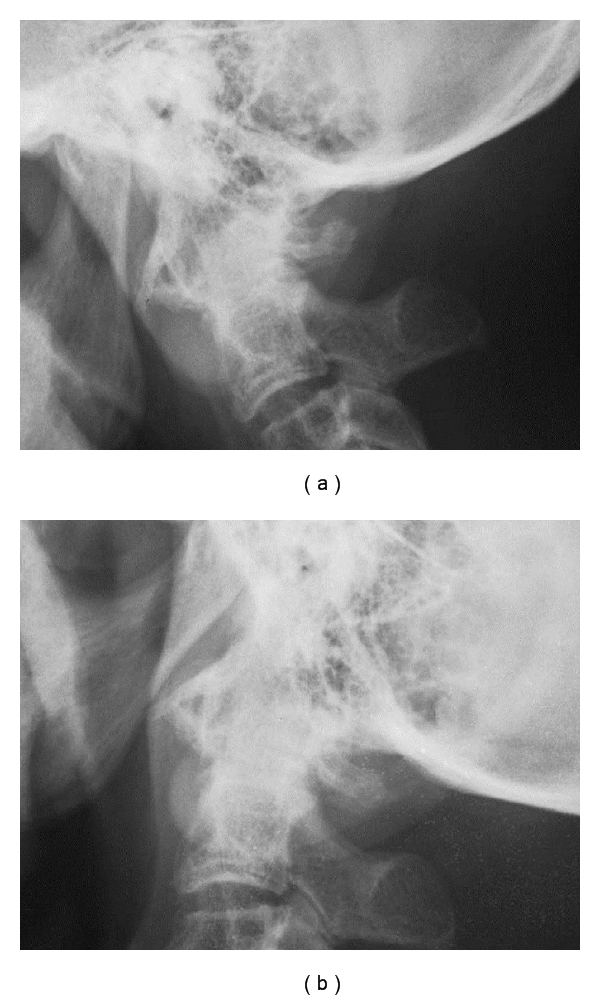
Postoperative dynamic lateral plain radiographs. Flexion (a) and extension (b) view. Atlantoaxial subluxation had not progressed.
